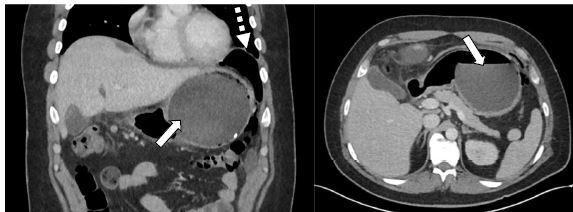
Computed tomography of the abdomen and pelvis, coronal view (left) and axial view (right), with intravenous contrast demonstrating intraluminal gastric balloon (solid arrow) with perforation and free air in the left upper quadrant (dashed arrow).
